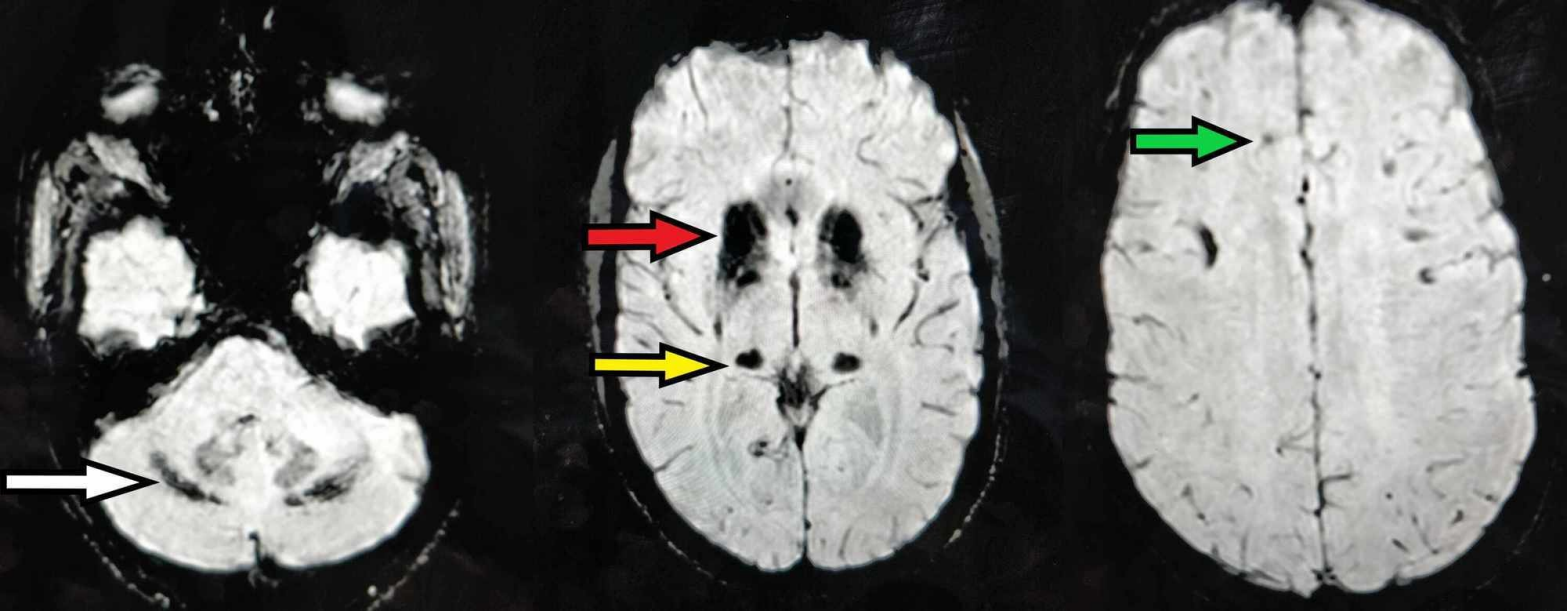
Axial sections of the SWI sequence of MRI brain showing bilateral symmetrical calcification SWI, susceptibility-weighted imaging; MRI, magnetic resonance imaging The calcification is evident as blooming, predominantly in bilateral basal ganglia (red arrow) and thalami (yellow arrow). Smaller lesions are also seen in the frontal cortex (green arrow) and cerebellum (white arrow).
